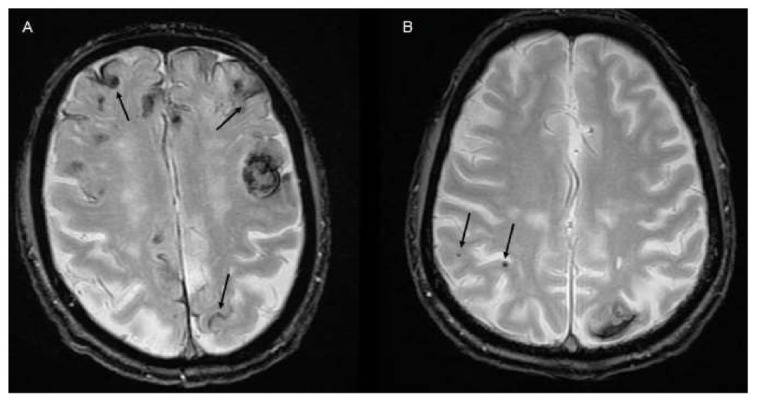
Small vessel disease markers. (A) MRI with evidence of lobar ICH and diffuse cortical superficial siderosis (arrows). (B) MRI with evidence of lobar ICH and lobar cerebral microbleeds (arrows).
